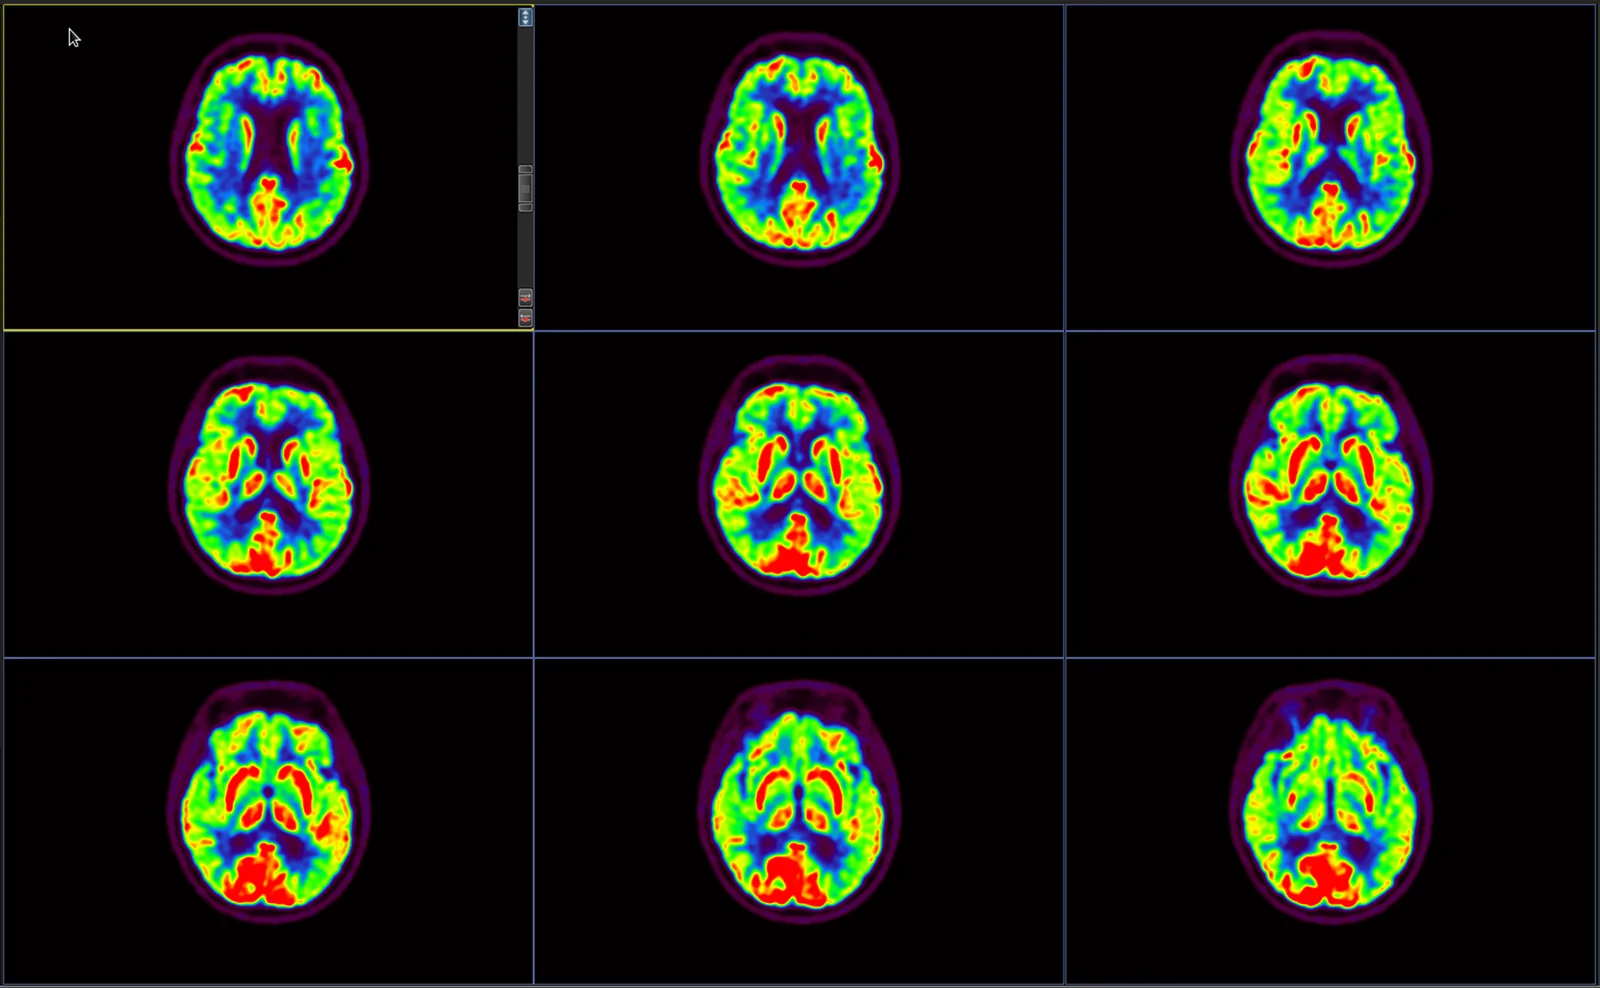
Brain ^18^F-FDG PET imaging. Brain positron emission tomography (PET) scan showing a pattern of diffuse cortical hypometabolism with selective metabolic sparing of the basal ganglia, compatible with metabolic/iatrogenic encephalopathy.
